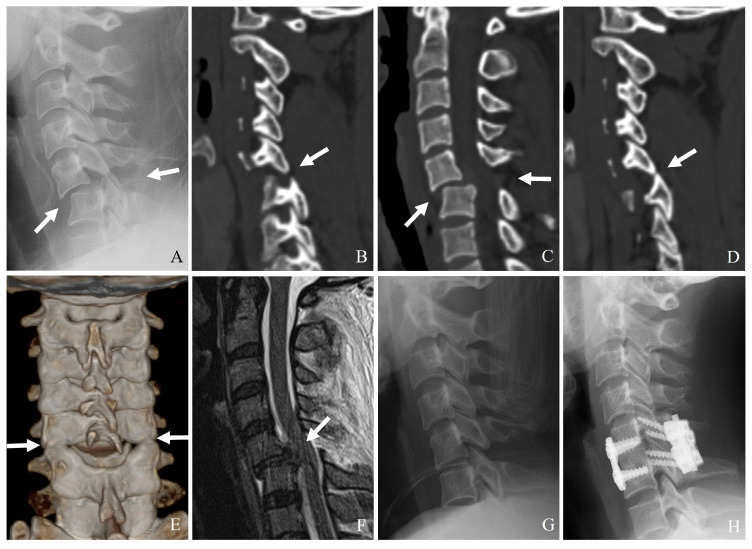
Plain X-ray (A), computed tomography (B–E), and magnetic resonance imaging (F) reveal bilateral facet dislocation and traumatic disc herniation at C5–6 (white arrows). Successful closed reduction with skull traction was achieved (G). The patient underwent combined anterior cervical discectomy, fusion, and posterior fixation of C5–C6 (H).
